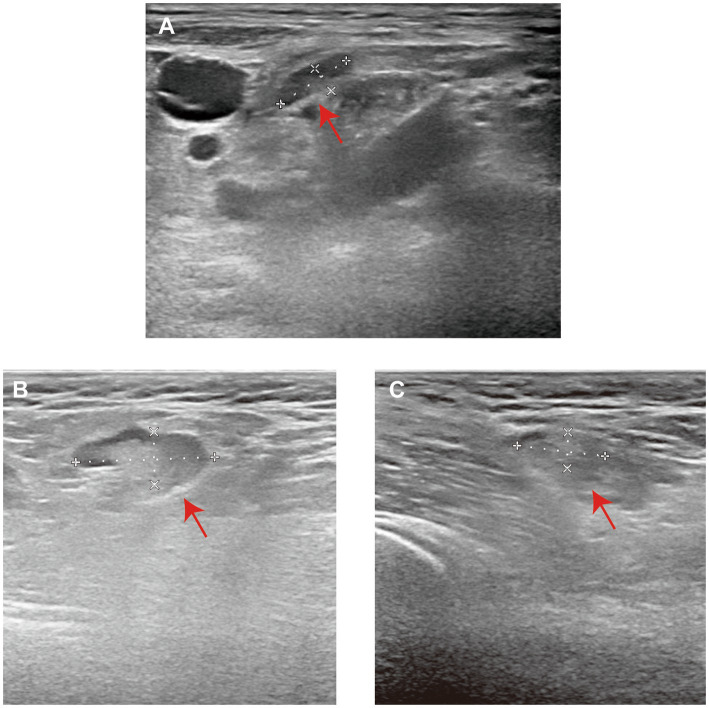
(A) Lymph nodes in the left supraclavicular fossa under Color Doppler ultrasound. (B) Lymph nodes in the left axilla under Color Doppler ultrasound. (C) Lymph nodes in the right axilla under Color Doppler ultrasound.
